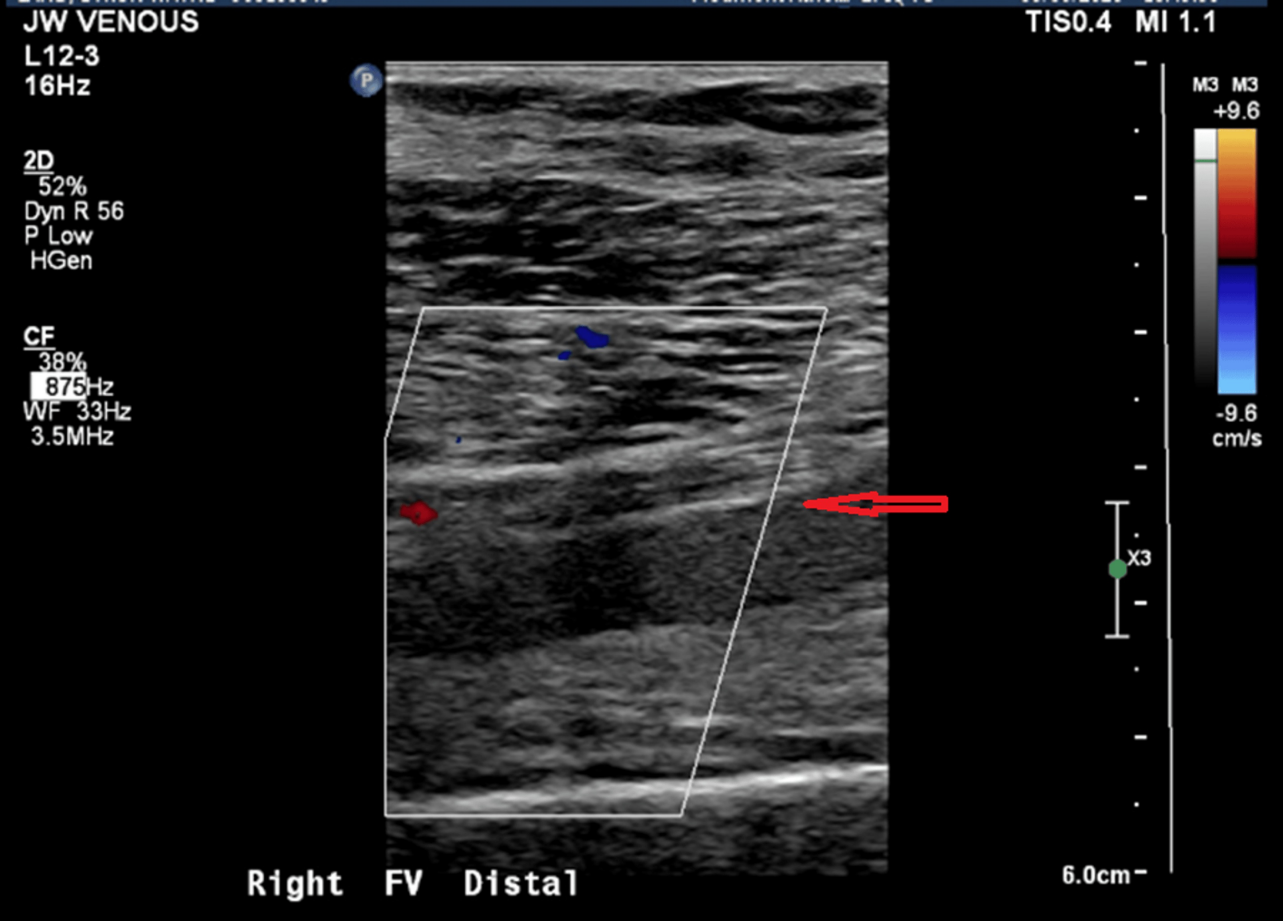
Ultrasound image of right distal femoral vein thrombosis Compression ultrasound of the right thigh showing a non-compressible, dilated right distal femoral vein (arrow) with an acute thrombus, consistent with acute deep vein thrombosis.
